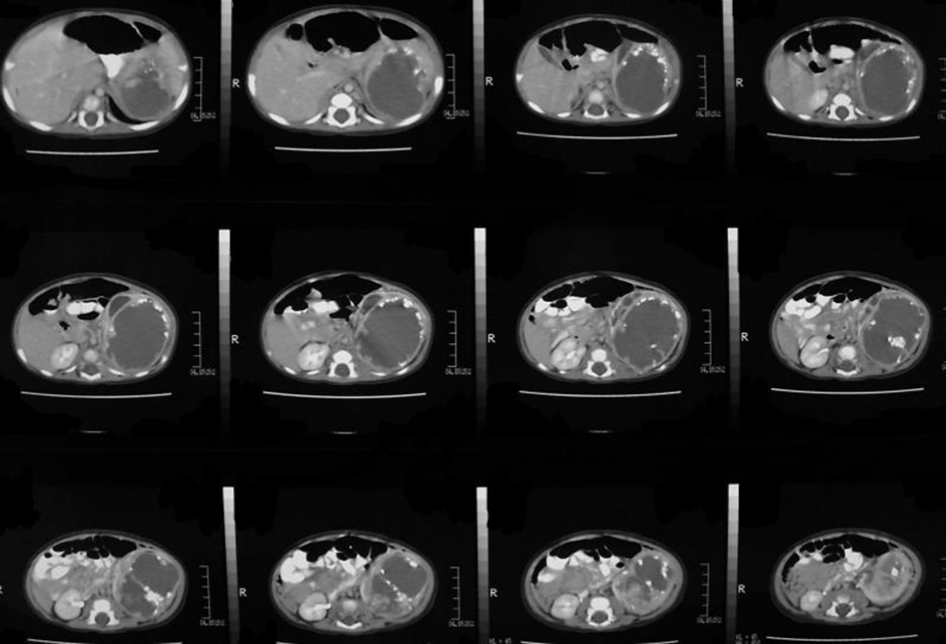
Helical CT examination of heterogeneous, cystic renal mass within the left retroperitoneal site shows peripheral fatty tissue deposits with low attenuation values.
